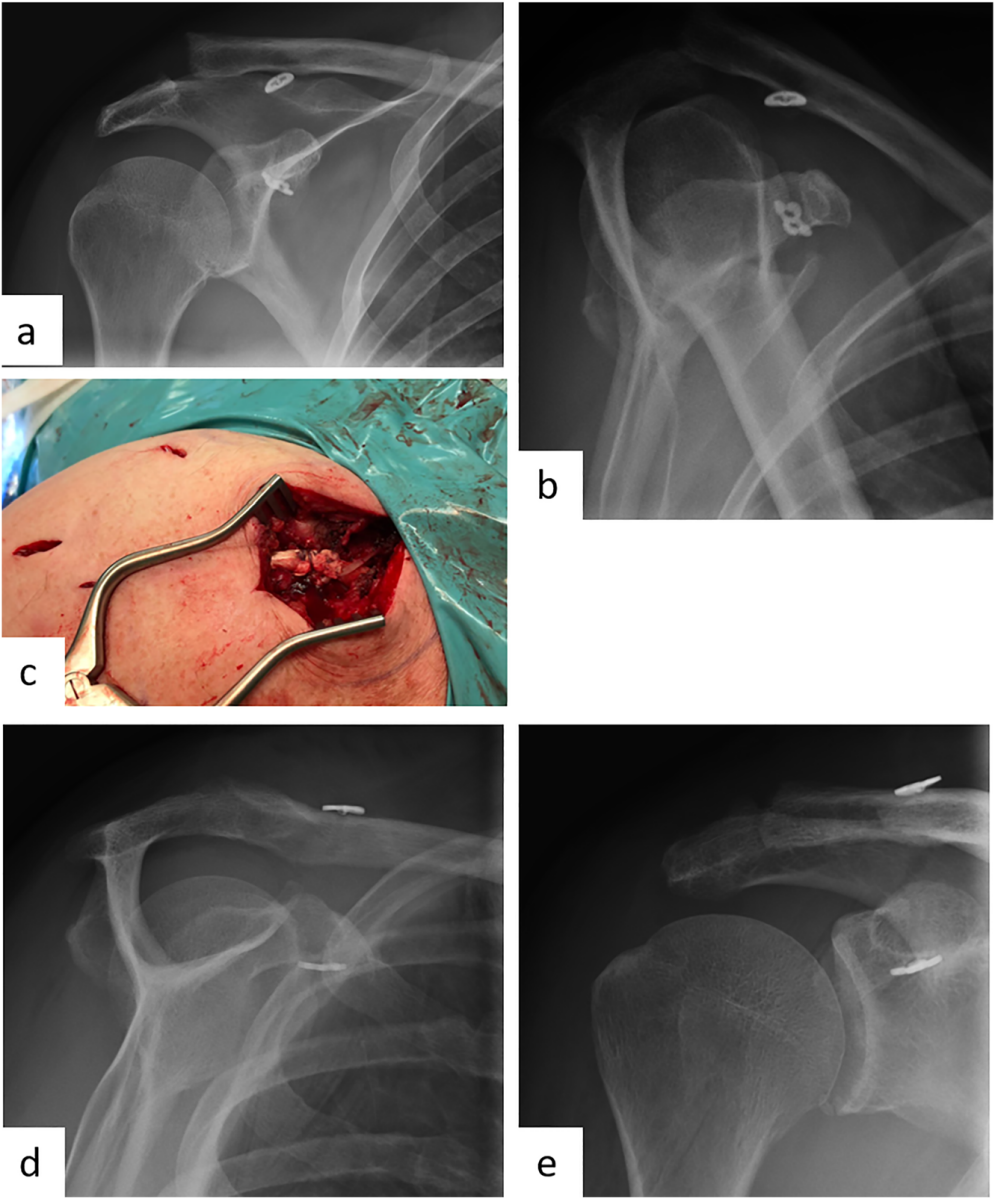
Male patient (55 y), right shoulder, chronic acromioclavicular joint instability with loss of reduction after surgical displacement of an acute Rockwood 3B injury 3 months ago (preoperative X-ray): a – Zanca stress radiograph with 10 kg weight, b – Alexander radiograph, c – arthroscopically supported vertical and horizontal stabilization with a TightRope and an additional autologous Hamstring-tendon augmentation, postoperative X-ray: d – Outlet view, e – a.p.
